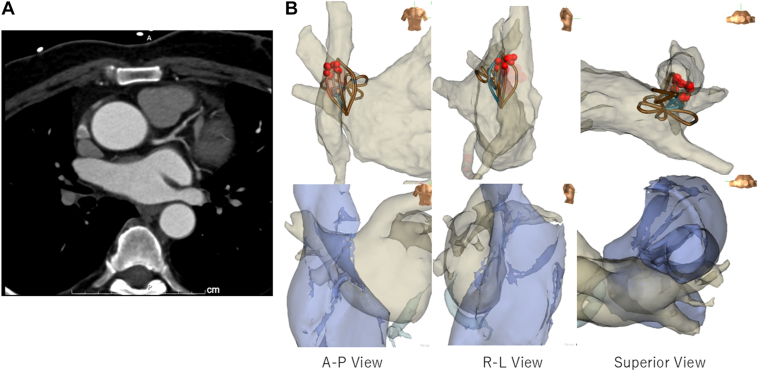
Anatomical relationship between the SVC and RSPV and ablation sites. A: Contrast-enhanced CT demonstrates the close proximity of the SVC and RSPV. B: Upper panels: 3-dimensional electroanatomic map shows PFA applications delivered in basket and flower configurations at the RSPV. *Red tags* denote RF applications. *Red*-shaded regions represent projection mapping of points located within 5 mm of the third electrode on the PFA catheter. Lower panels: 3-dimensional CT reconstruction; the *blue chamber* is the RA, and the *white-gray chamber* is the LA. A-P = anteroposterior; CT = computed tomography; LA = left atrium; PFA = pulsed-field ablation; RA = right atrium; RF = radiofrequency; R-L = right-to-left; RSPV = right superior pulmonary vein; SVC = superior vena cava.
